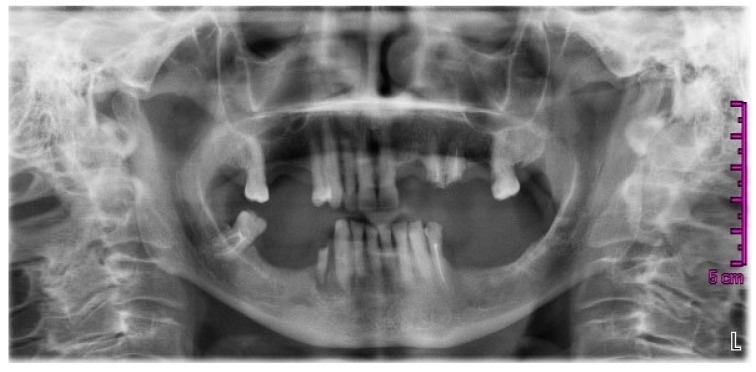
Orthopantomography dated March 2014.
